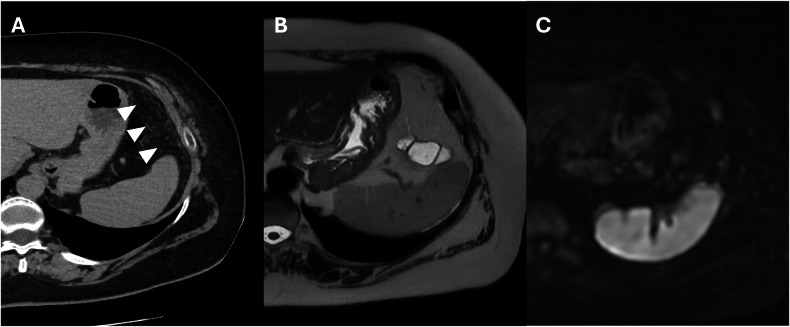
Colon adenocarcinoma with peritoneal carcinomatosis in a 74-year-old woman. A CT delayed phase, axial plane. Relevant diffuse implants with micronodular pattern in the left hypochondrium (arrowheads). MRI T2w and DWI (b = 800 mm^2^/s) (B, C) axial plane, no micronodular implants are visibleThe nodular pattern is characterized by a nodular lesion greater than 5 mm in diameter that might be solid or cystic, depending on the primary tumor (Fig. 4) [26].
